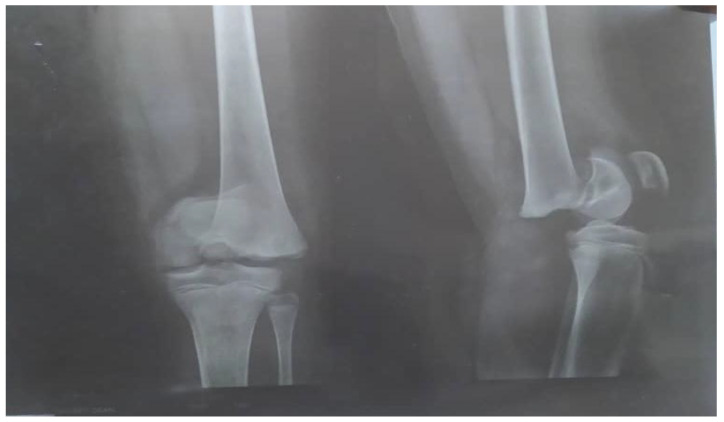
Plain X-ray of the left knee with mechanical non-union fracture.
